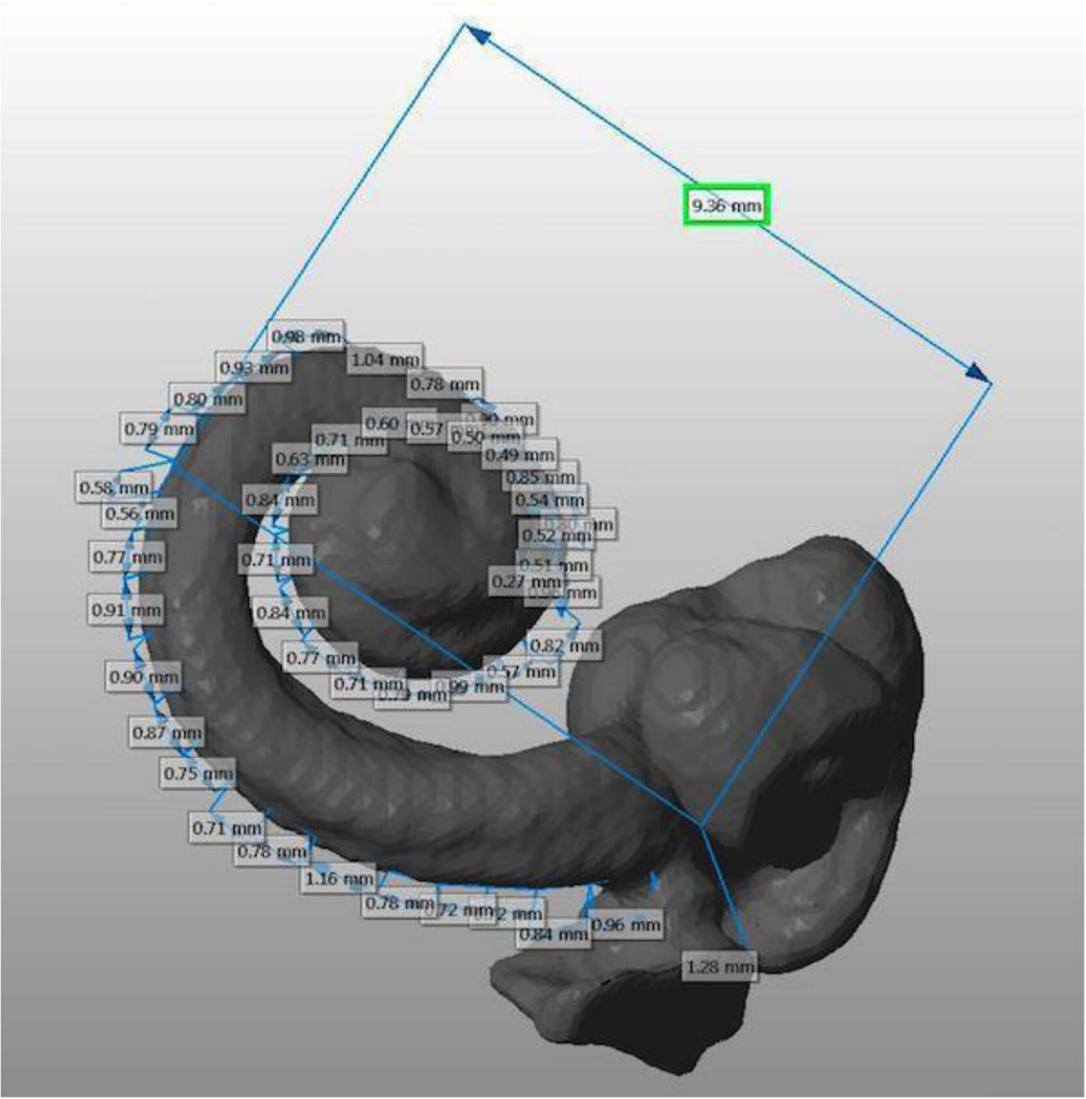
3D mesh model of cochlea segmented from CBCT scan used to measure cochlear duct length and basal diameter. Incremental measurements displayed in millimeter from the round window, along the lateral wall of cochlear duct length, for the first two turns of the cochlea (360 and 720 degrees)
